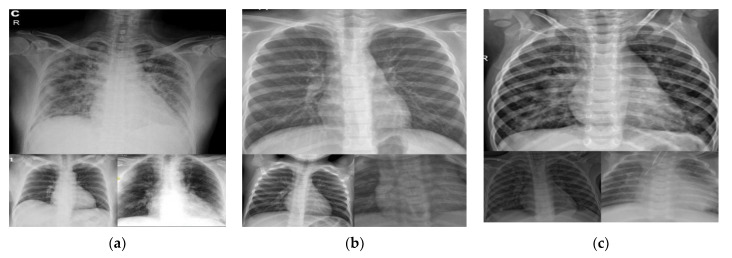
Sample X-ray images (a) COVID-19 lung X-ray images; (b) Healthy chest X-ray images; (c) Pneumonia chest X-ray images.
